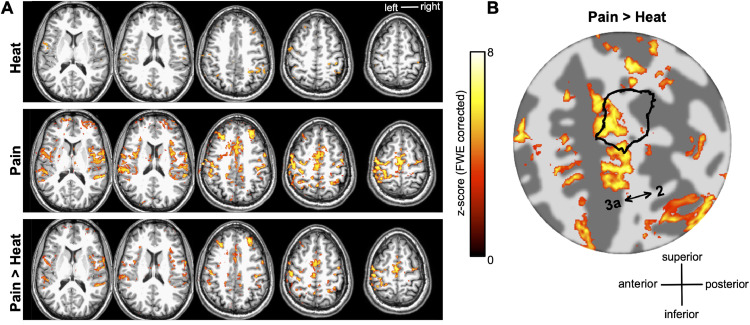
(A) Cortical activation in response to innocuous thermal (hot) stimulation (Heat, top row) of the thenar eminence compared to thermonoxious stimulation (Pain, middle raw) on the same skin surface (subject 1) overlaid onto structural T1-weighted space. The contrast Pain>Heat (bottom row) showing areas where thermonoxious BOLD evoked responses are larger than BOLD responses induced by a change in temperature is very similar to the Pain contrast (both displayed at *z*>3.08 after FWE correction, while the Heat contrast map is uncorrected). (B) Statistical map for contrast Pain>Heat overlaid onto a flattened representation of the central sulcus. Notice that the activation extends anteriorly and inferiorly with respect to delineation of the tactile digit territory (shown by the black solid outline).
